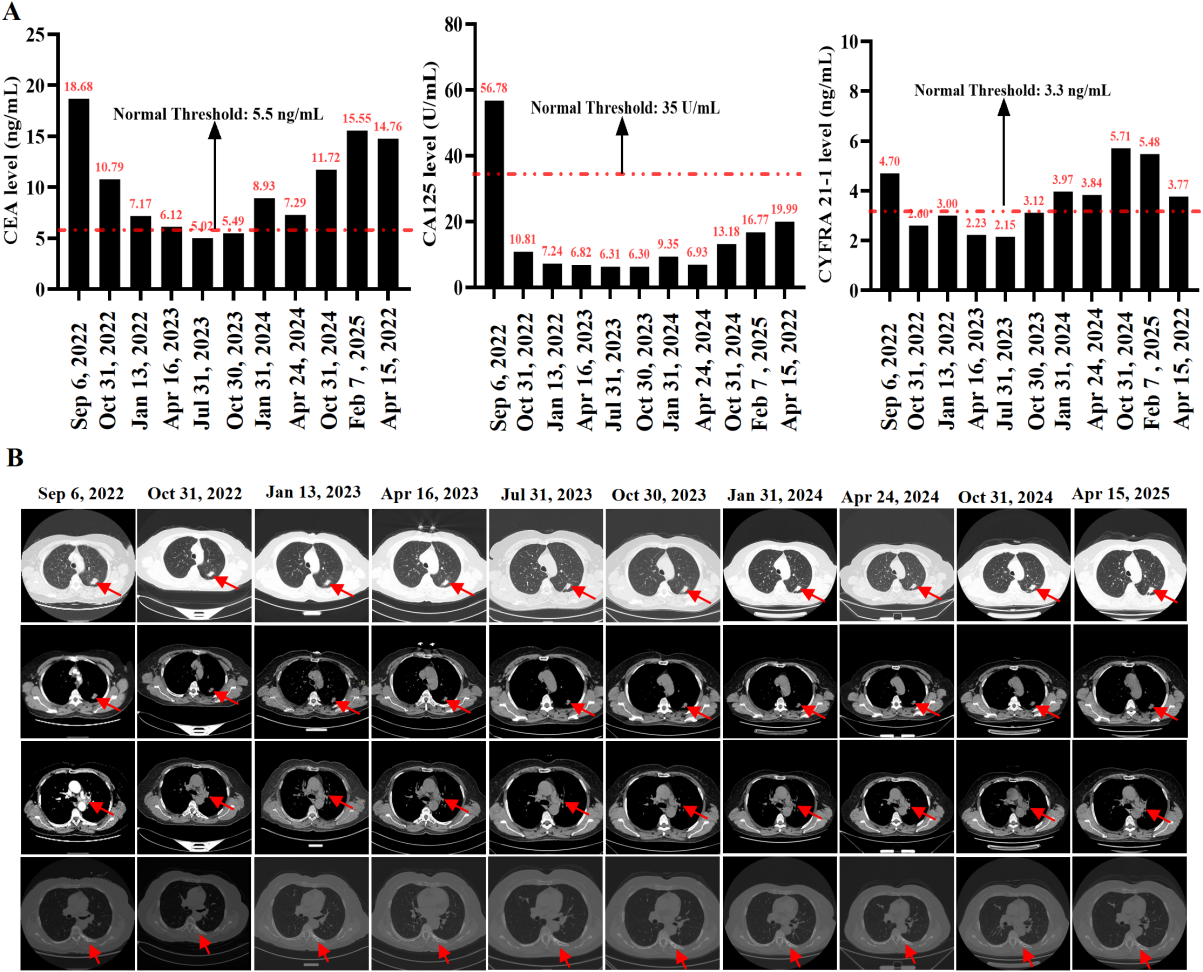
(A) Serial measurements of serum tumor markers including carcinoembryonic antigen (CEA), carbohydrate antigen 125 (CA125), and cytokeratin-19 fragment (CYFRA21-1) from baseline to April 15, 2025. (B) Representative serial chest computed tomography (CT) images from baseline (September 6, 2022) through follow-up (April 15, 2025). Red arrows indicate the primary lung lesion.
